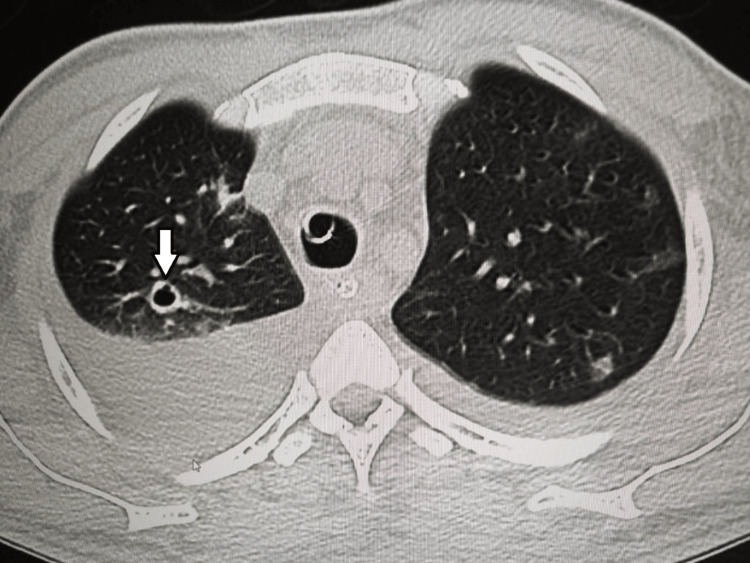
A CT scan of the chest The scan shows a right upper lobe cavitary nodule (white arrow) with left lung ground-glass nodules and bilateral pleural effusion.
